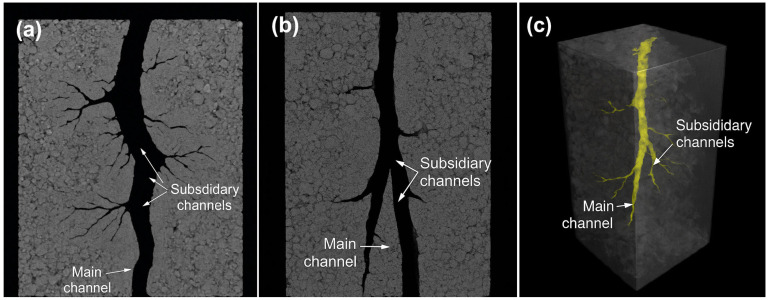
μ-CT reconstruction of channel structures induced by nonionic penetrant. (a) Transverse cross-section showing a main channel and numerous radial subsidiary branches forming a sparse peripheral network; (b) Longitudinal view displaying asymmetric bifurcations and multiscale channel distributions; (c) 3D rendering visualizing a central backbone structure with capillary-like peripheral branches.
